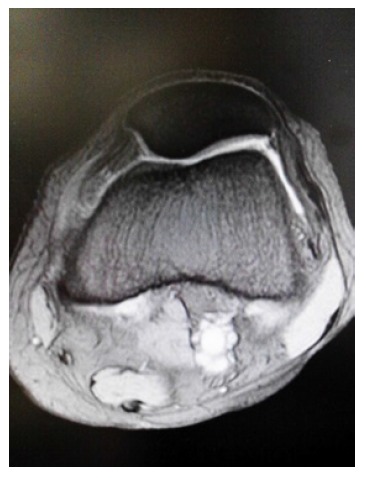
Magnetic resonance imaging showing a slack and torn medial patellofemoral ligament at the femoral attachment.
